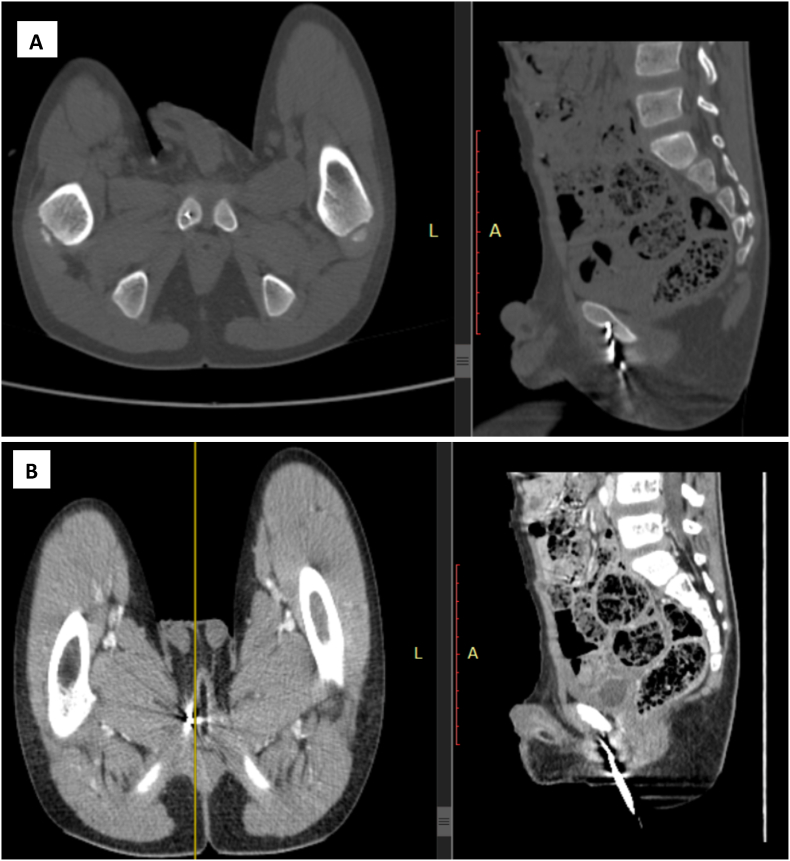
Axial and Sagittal views of Pelvic CT scan, (A) bone window which shows hyperdense lesion in the perineal soft tissue with metallic artifacts. (B) postcontrast view of the same image.
